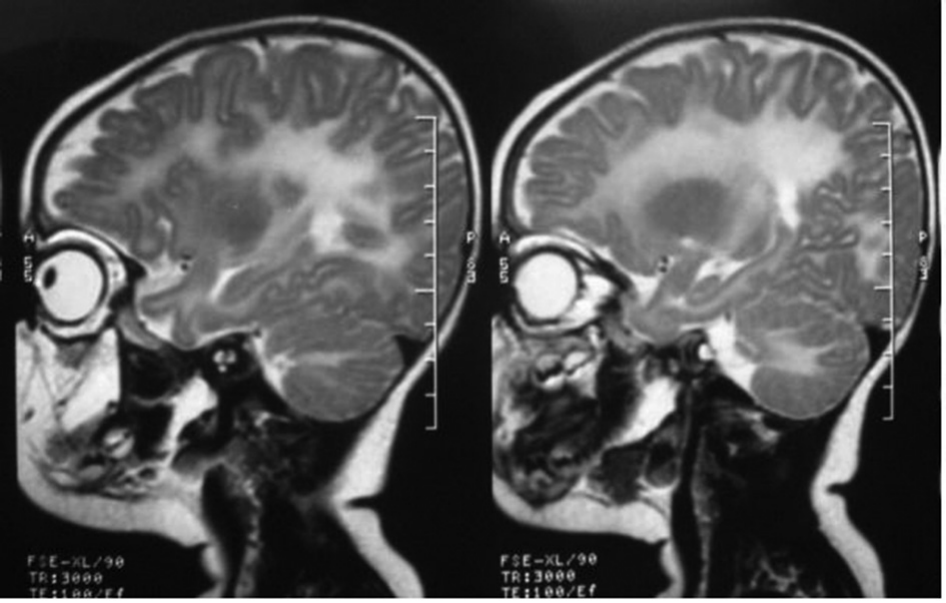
A ten-month-old girl with developmental delay. Sagittal T2 weighted MRI reveals diffuse increased signal intensity in the cerebral and cerebellar white matter. U fibers are also involved.
